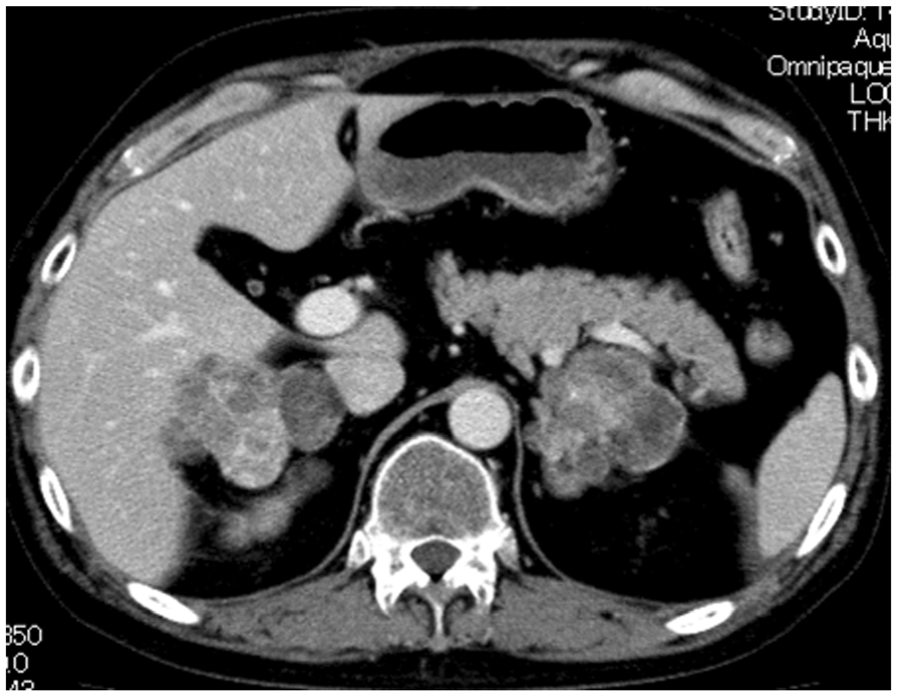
Abdominal computed tomography in patient 2 showing bilateral multinodular adrenal enlargement with maximum diameters of 8.5cm and 10.5cm on the right and left sides, respectively.
